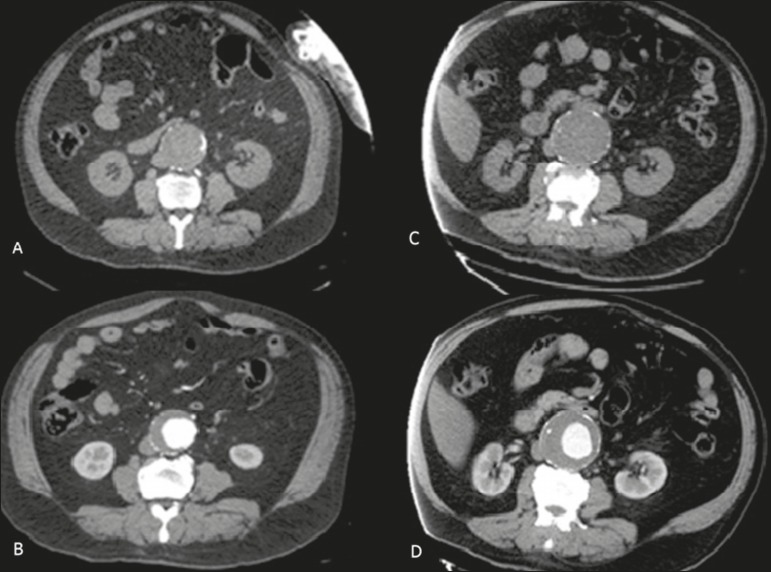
Unenhanced CT scans of a 73-year-old male patient in whom the maximum
